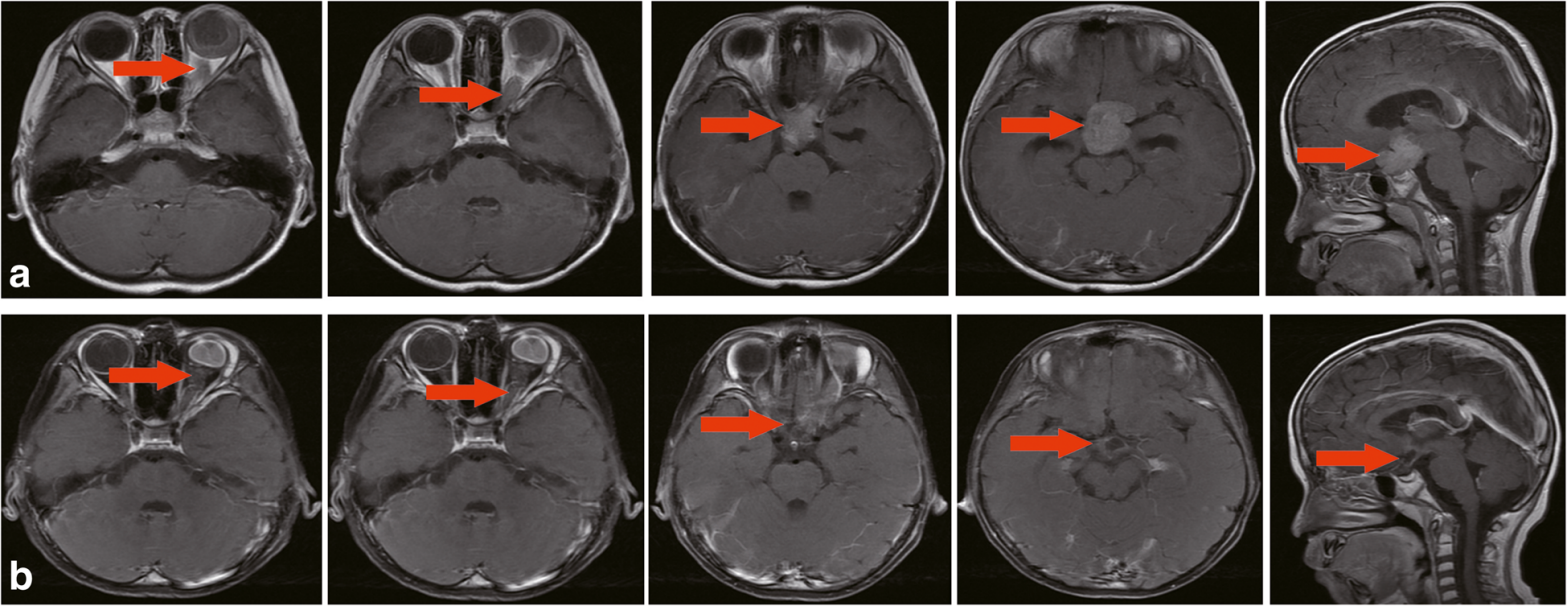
Contrast-enhanced MRI images of tumor regression and attenuated meningeal enhancement after chemotherapy. Coronal, and sagittal images show the mass in the supra sella cistern and enhanced signal of cerebral falx before chemotherapy (a), which were improved after chemotherapy (b). MRI: magnetic resonance imaging
